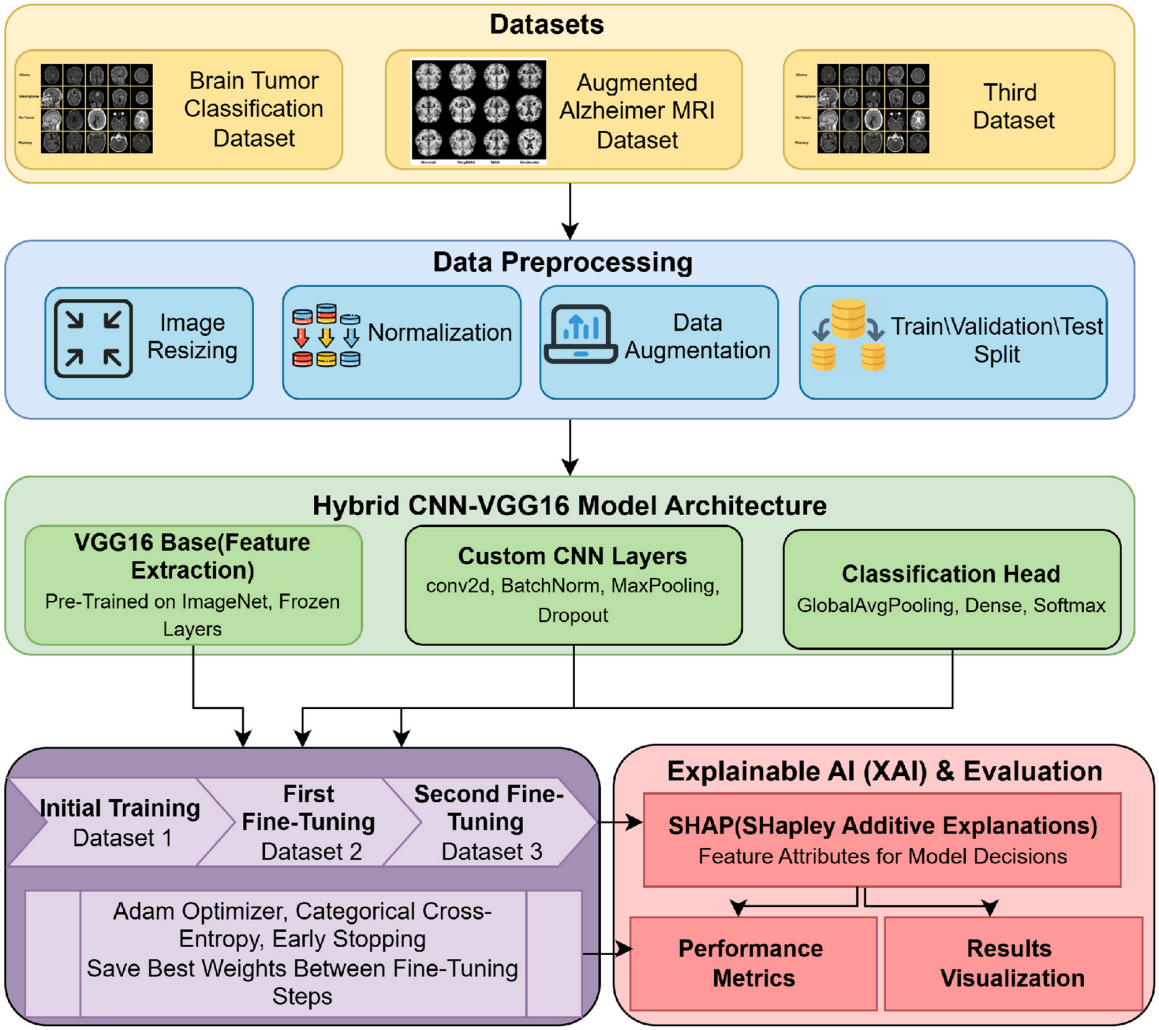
Hybrid CNN-VGG16 model with transfer learning and XAI for MRI classification.
